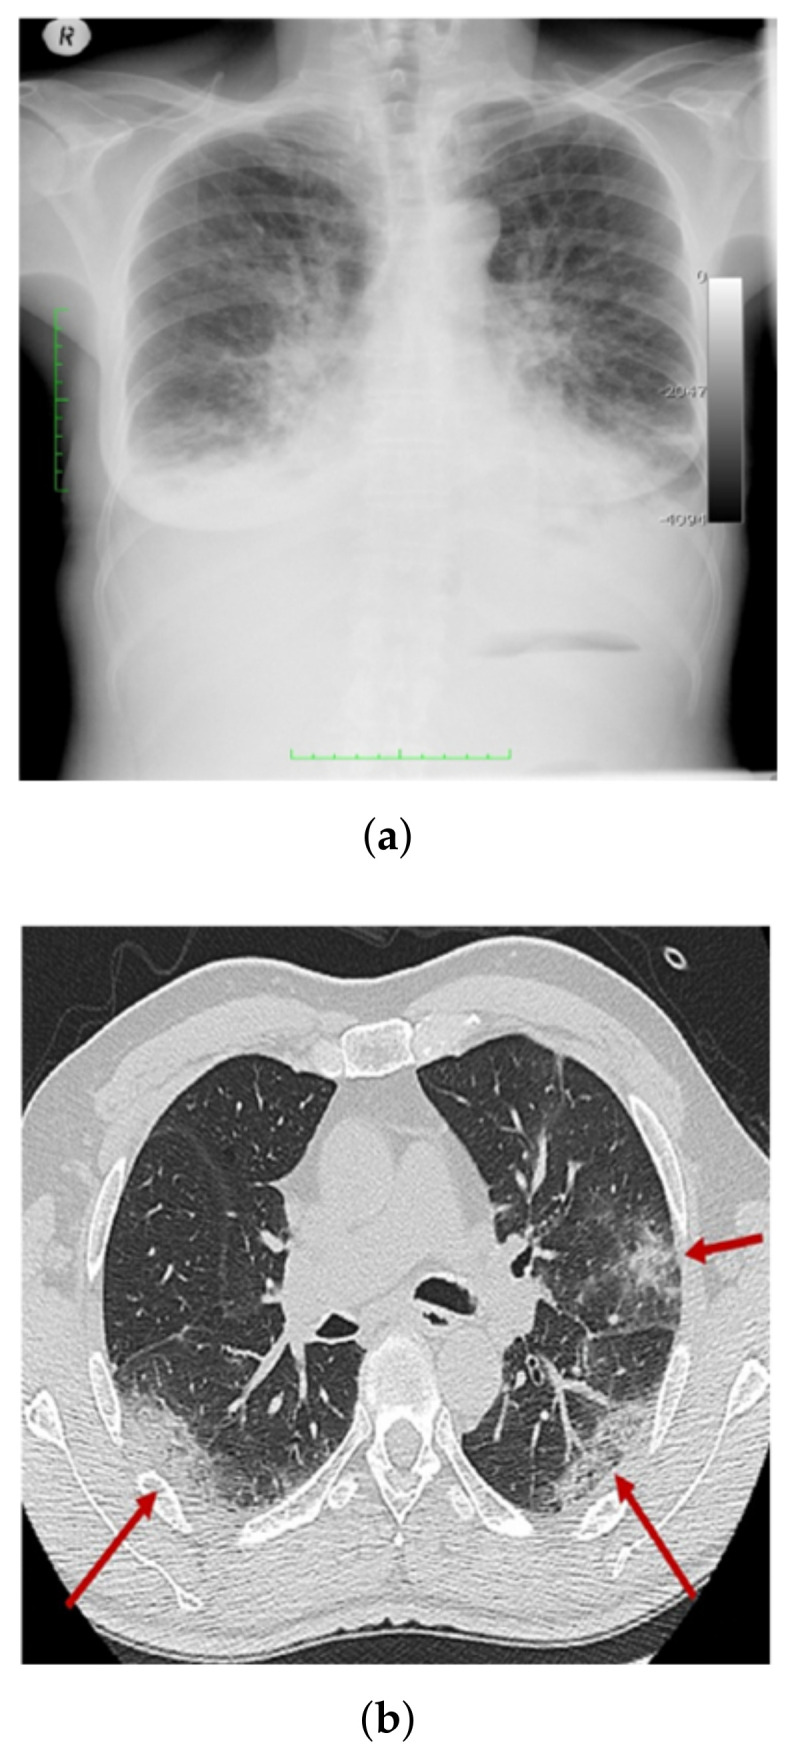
Samples of CXR images and CT images (a), and CXR image scan (b) CT image scan.
